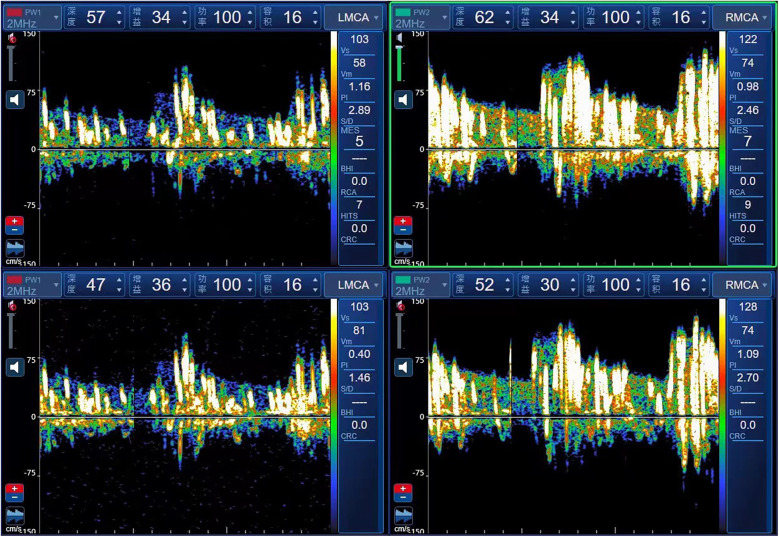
Positive contrast-transcranial Doppler ultrasonography test with grade III.
